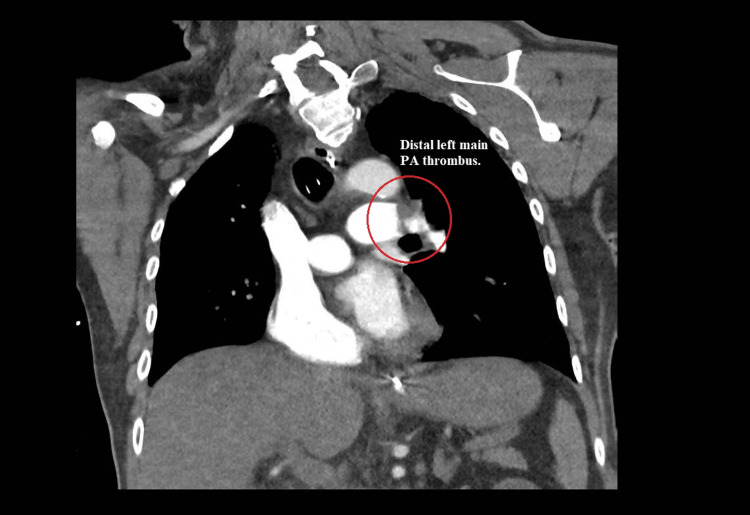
Coronal chest computed tomography angiography (lung window) demonstrating a filling defect in the distal left main pulmonary artery (circled region), consistent with an acute pulmonary embolus.
